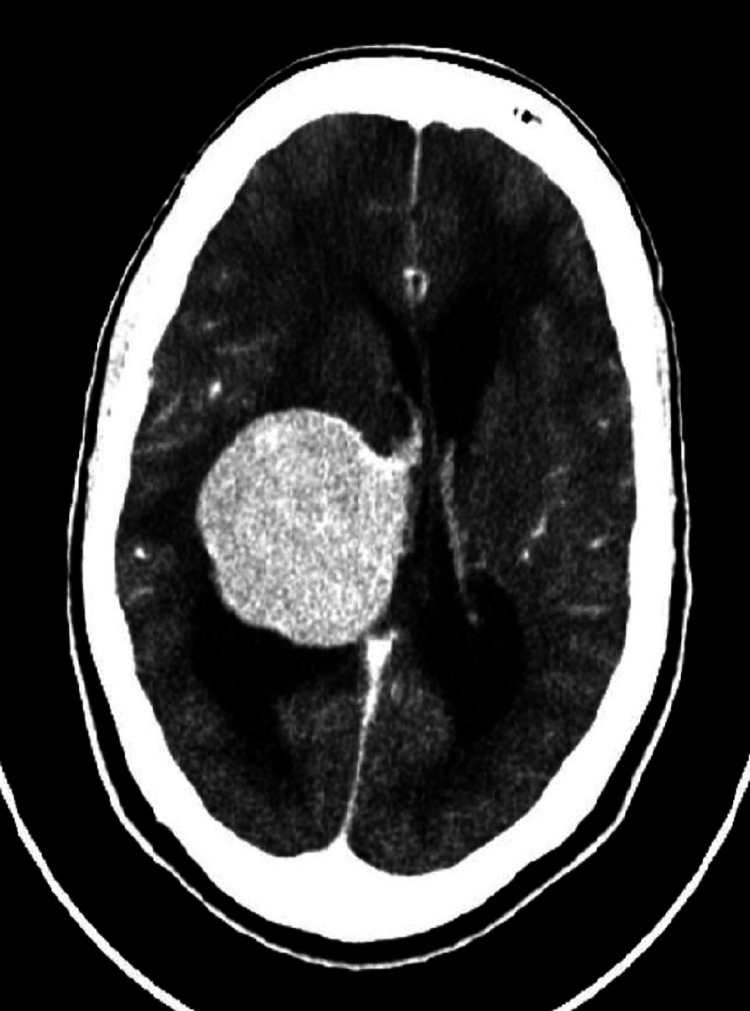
CT brain with contrast (axial view) demonstrating space-occupying lesion within the right lateral ventricle.
